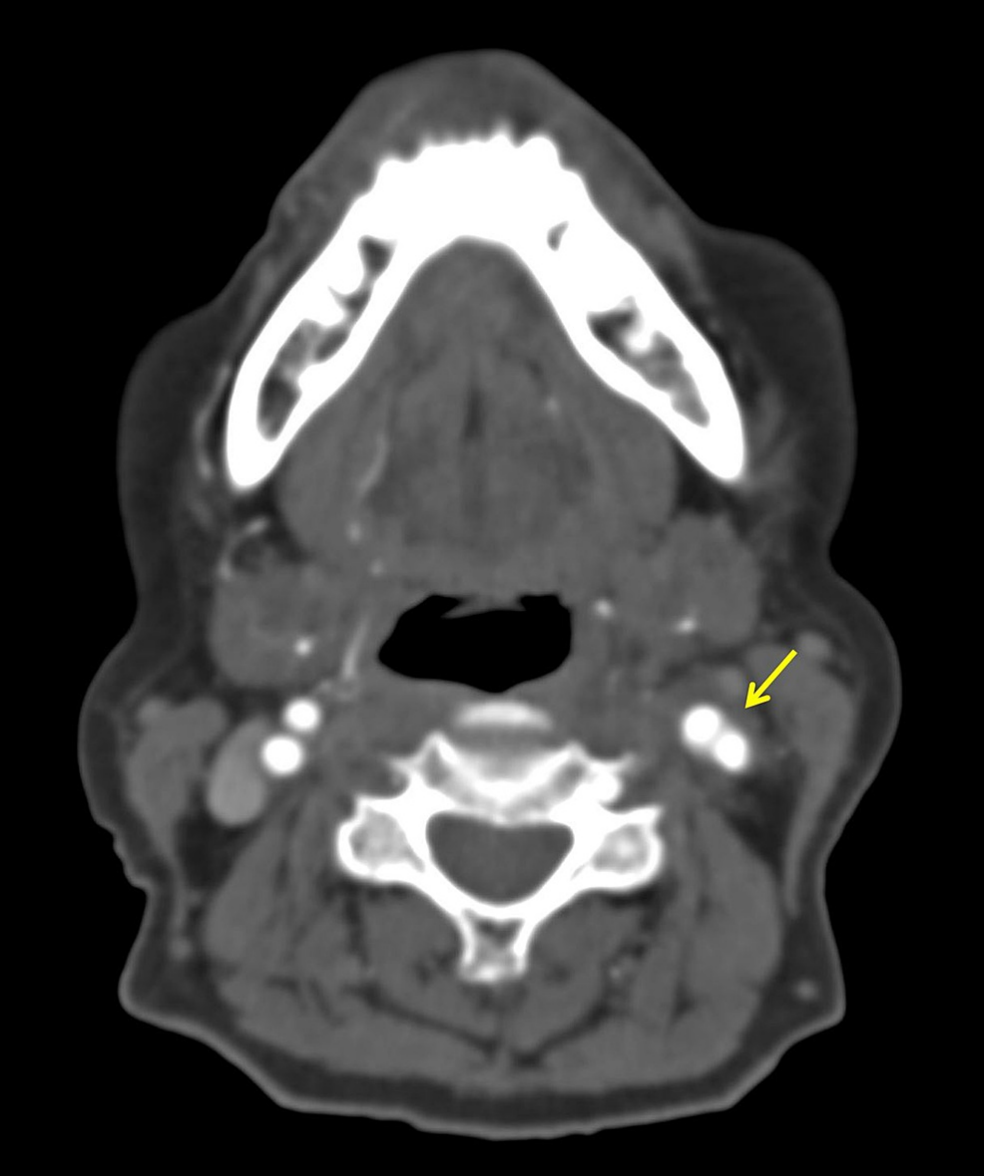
Axial contrast-enhanced CT image revealing the presence of enhancing inflammatory soft tissue (arrow) encircling the left carotid bifurcation.
